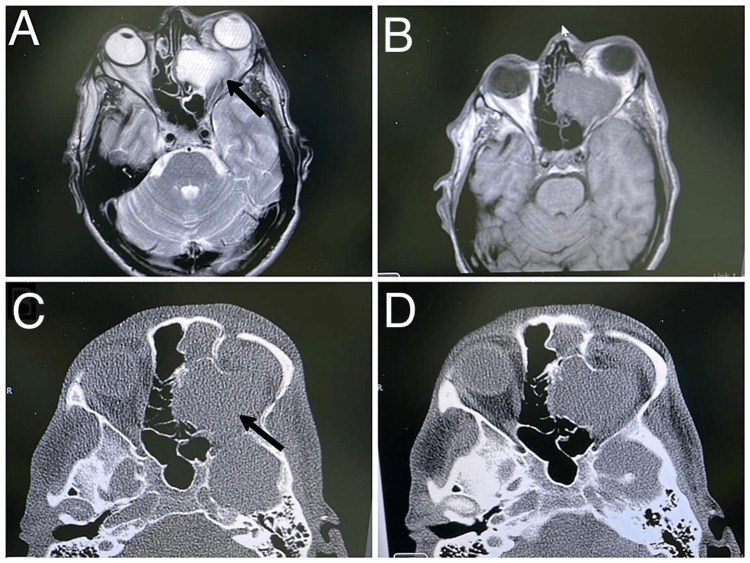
Case 6. (A,B) Left fronto-ethmoidal mucocele with obstructed left frontal drainage and associated with left unilateral proptosis (low signal intensity in T1 and high signal in T2); it is significantly encroaching upon the left orbital cavity, displacing and compressing left orbital structures without gross invasion; (C,D) CT scan showing left fronto-ethmoidal lobulated cystic lesion measures about 5 x 4 x 2.8 cm, with extending mainly to left retrobulbar space with associated left proptosis; there is associated expansion/remodelling and erosions of the left lamina papyracea and medial superior orbital wall.
